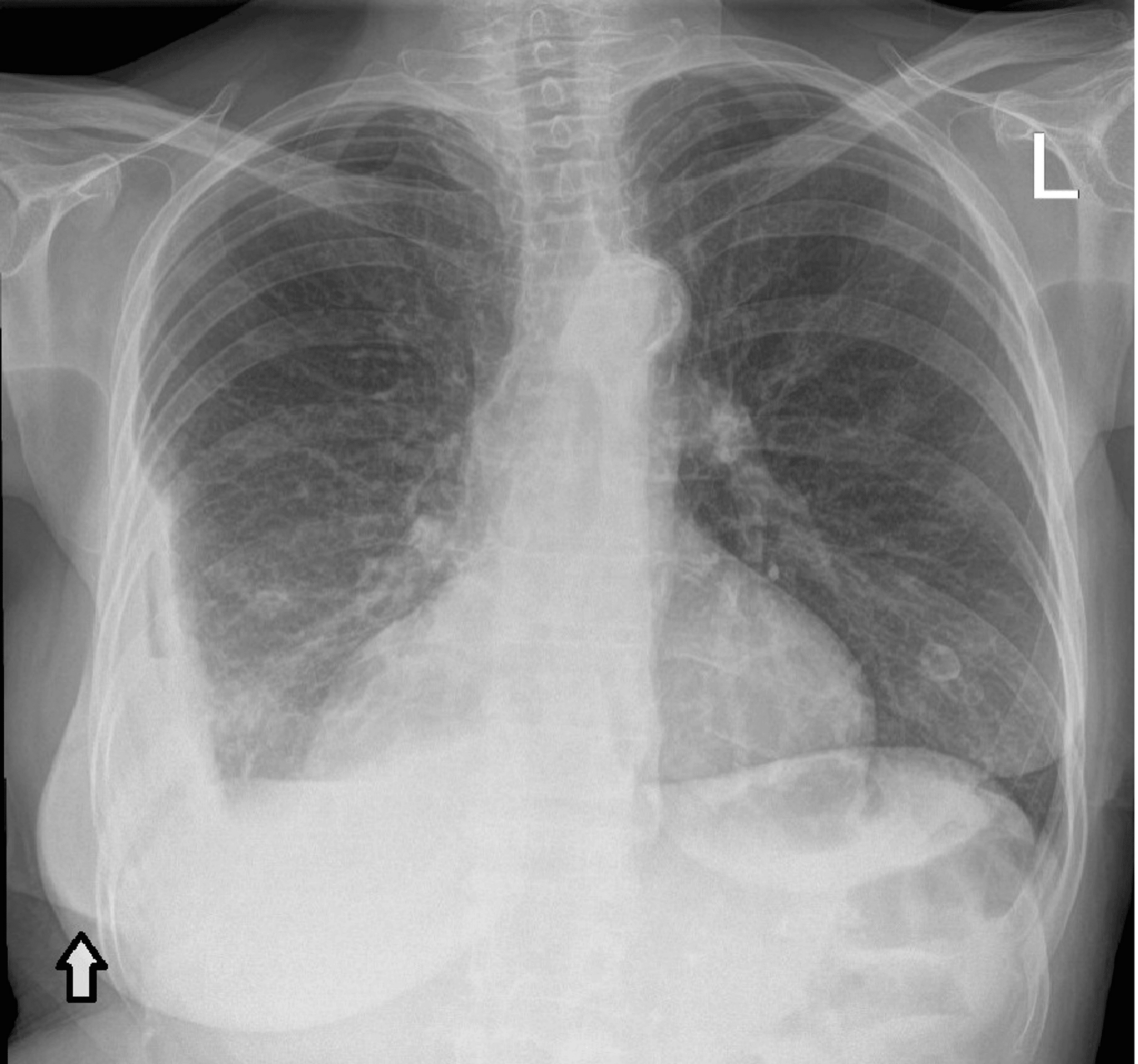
Chest X-ray following drain removal. Note the extension of the empyema into the thoracic wall
